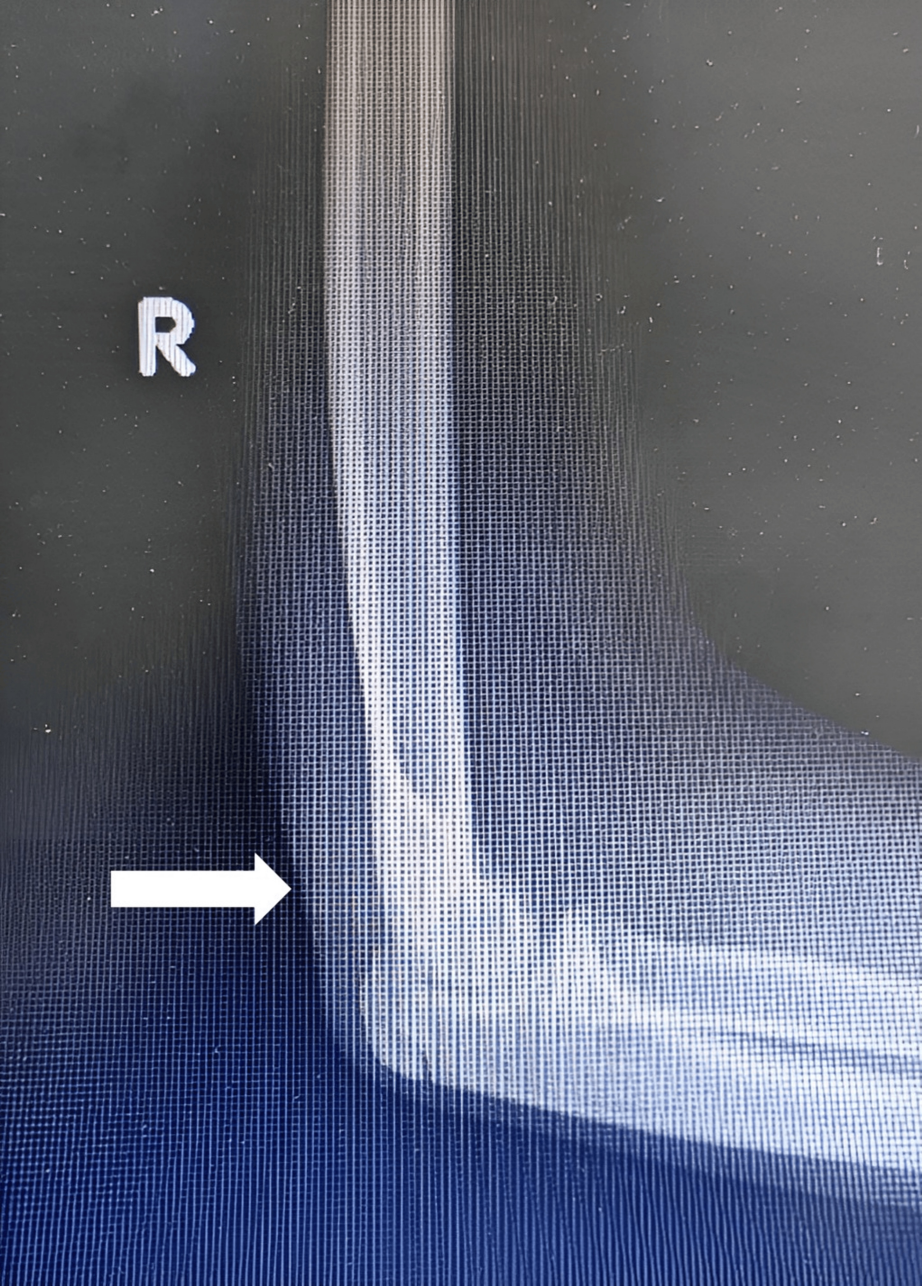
Post-resection lateral X-ray right elbow shows complete resection (arrow).
